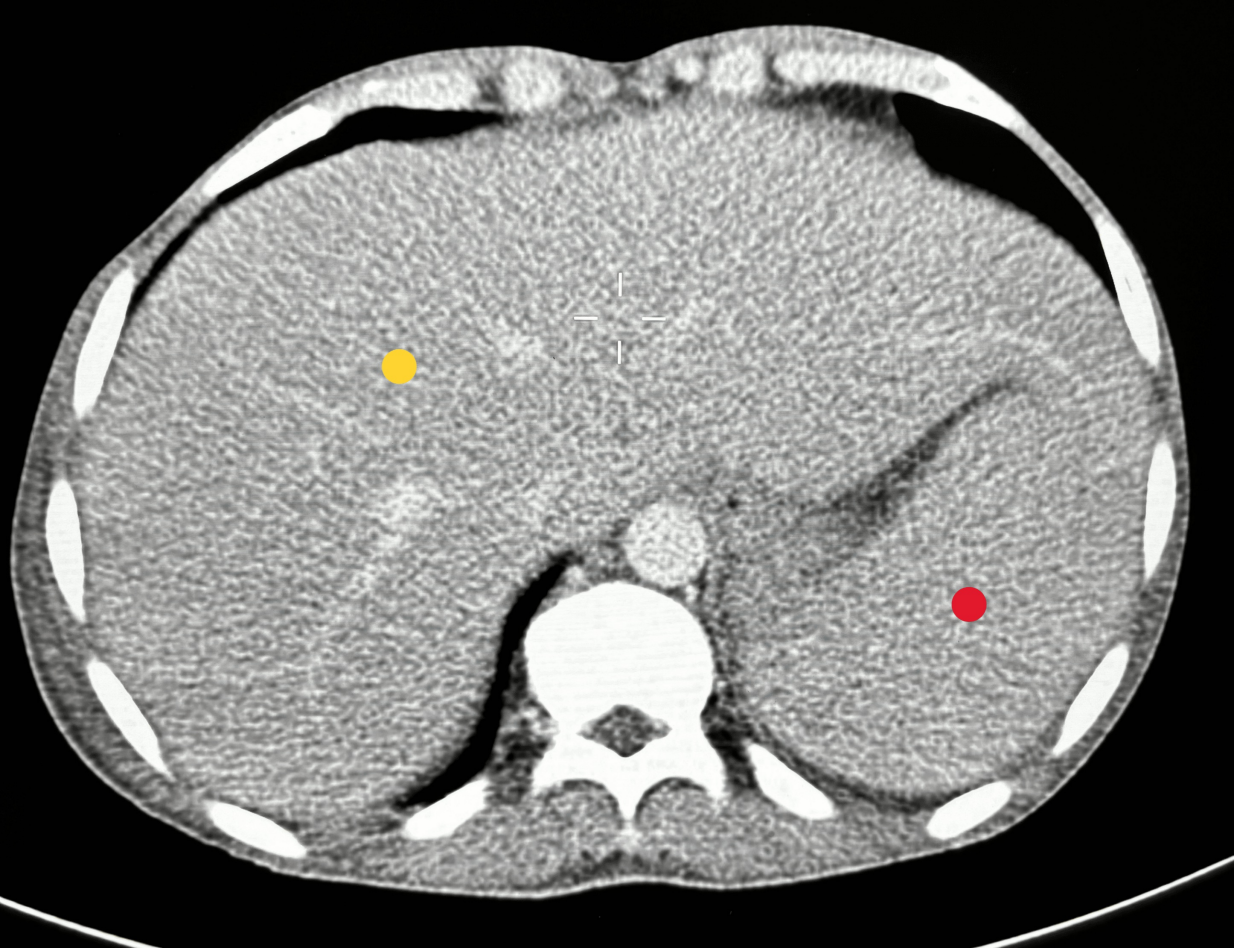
Axial CT of the abdomen demonstrating hepatosplenomegaly: hepatomegaly indicated by a yellow dot and splenomegaly indicated by a red dot CT: computed tomography
